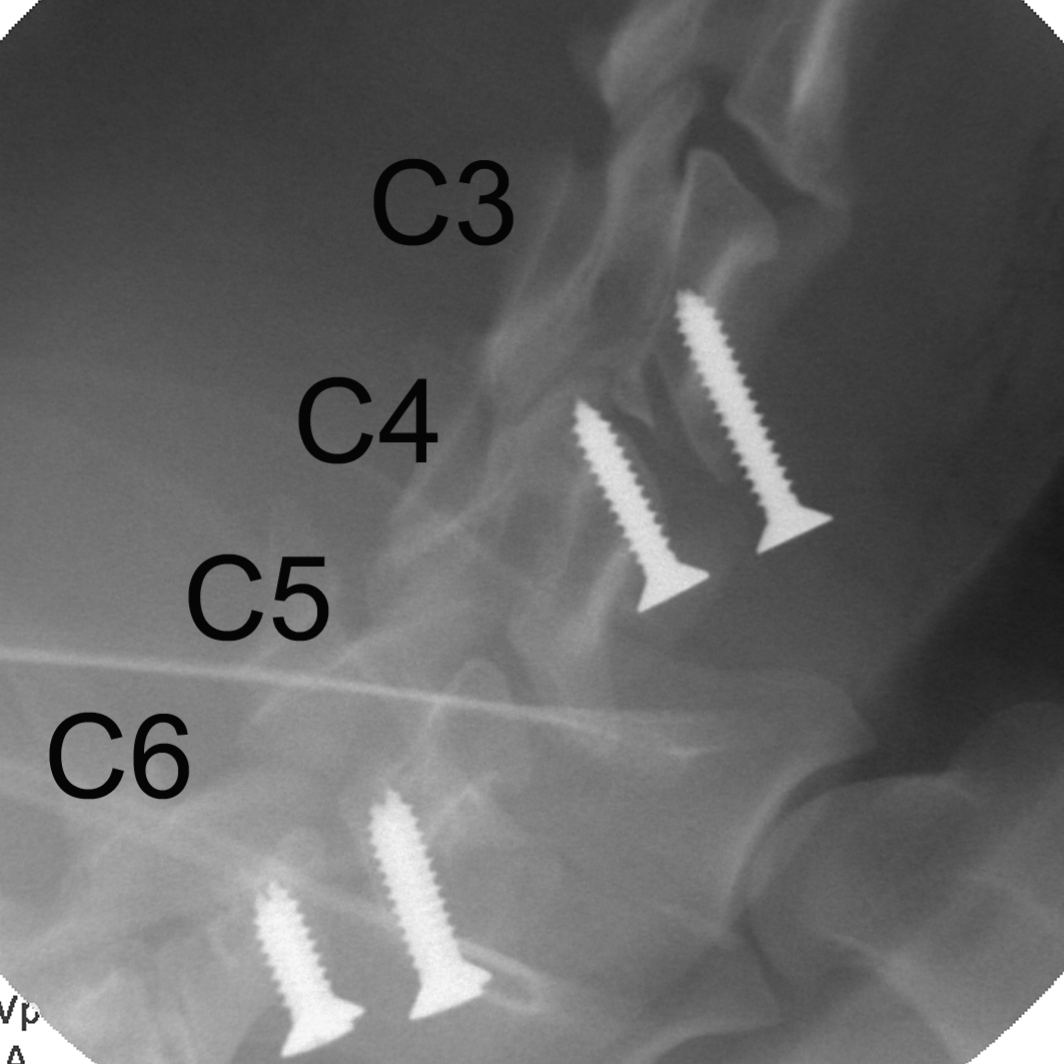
Postsurgical X-ray examination. PEEK spacers (radiolucent) were implanted and fixed with screws in the C3/4 and C5/6 intervertebral spaces.
